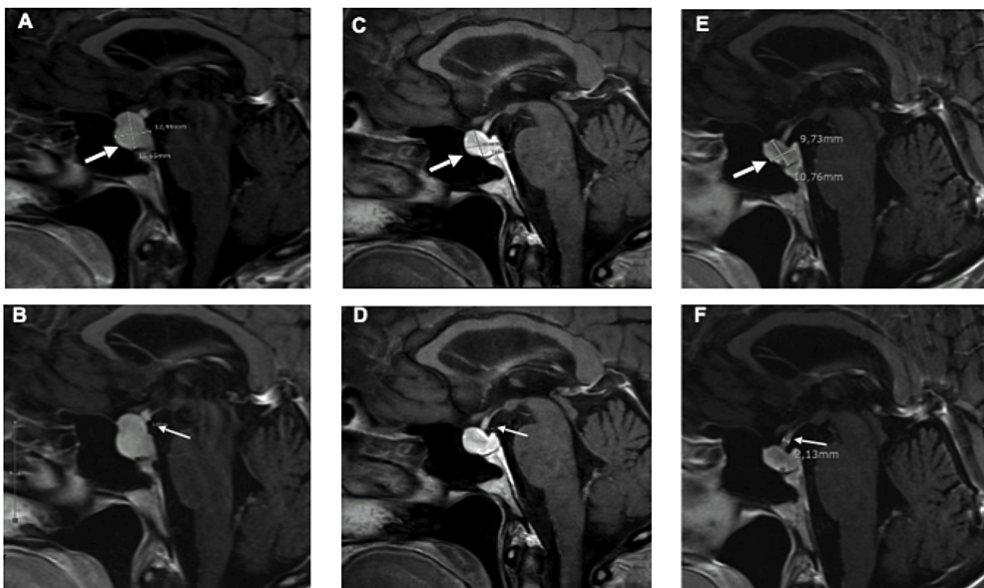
T1-weighted fluid-attenuated inversion recovery (FLAIR) sagittal MRI imaging features of the pituitary gland (thick arrows) and stalk (thin arrows) at diagnosis (A and B, respectively), three (C and D, respectively) and eight months after interruption of Pembrolizumab (E and F, respectively)
